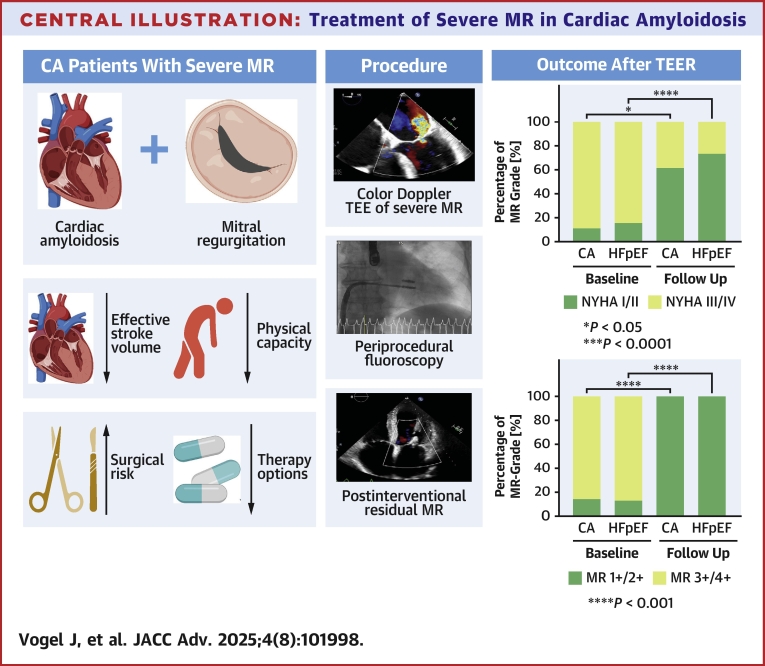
Treatment of Severe MR in Cardiac Amyloidosis (Left) Challenges in CA with MR. (Middle) TEER procedure. (Right) Outcome after TEER. CA = cardiac amyloidosis; HF = heart failure; MR = mitral regurgitation; TEER = transcatheter edge-to-edge repair; TEE = transesophageal echocardiography; HFpEF = HF with preserved ejection fraction.
